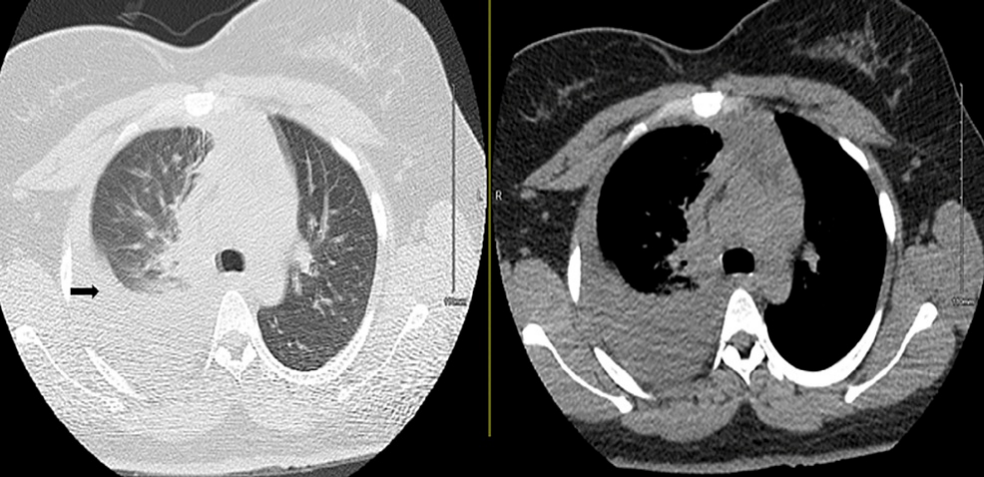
CT scan of chest done on the same day within 24 hours of initial presentation consistent with large right-sided pleural effusion (black arrow) CT: computerized tomography
